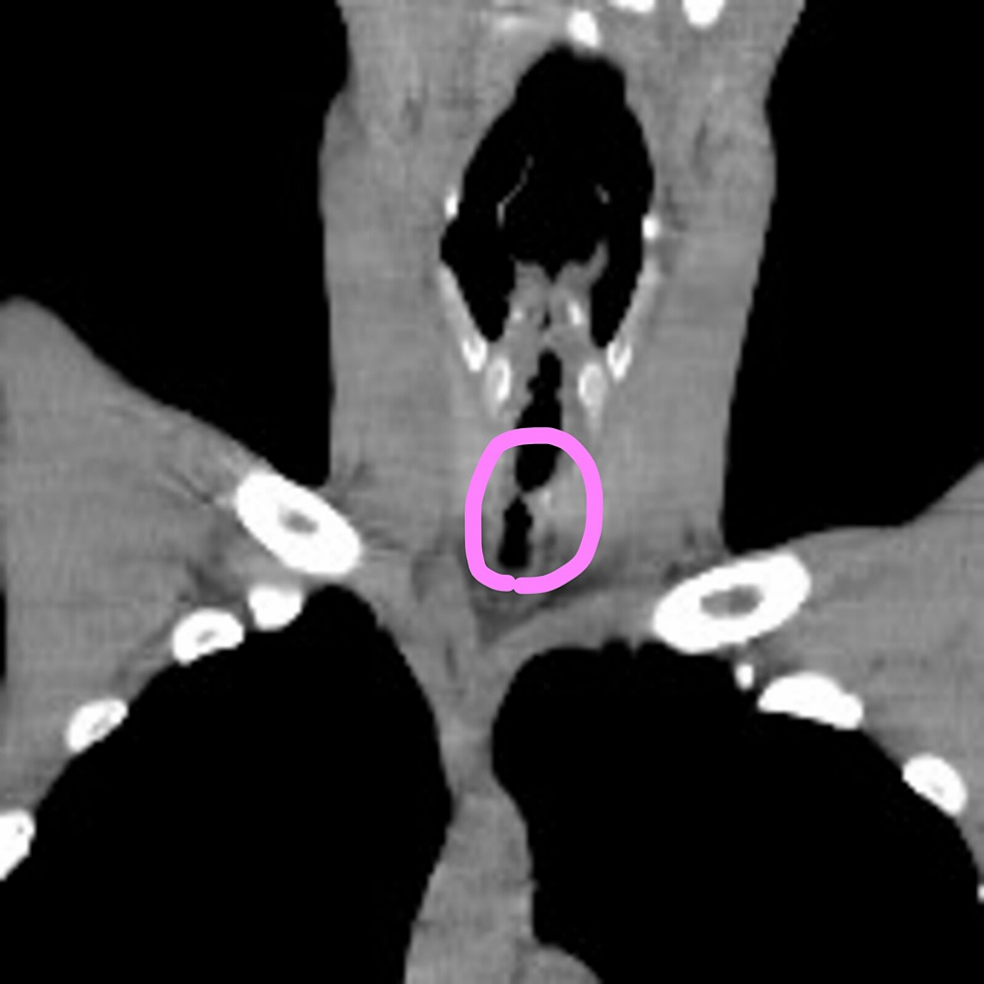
Cervical CT, lateral view. The patient’s medical records revealed that he had been diagnosed with benign subglottic stenosis three years earlier. At the time, the lumen was narrowed to 9 mm over a length of 46 mm, from the subglottis to the caudal side of the trachea.
